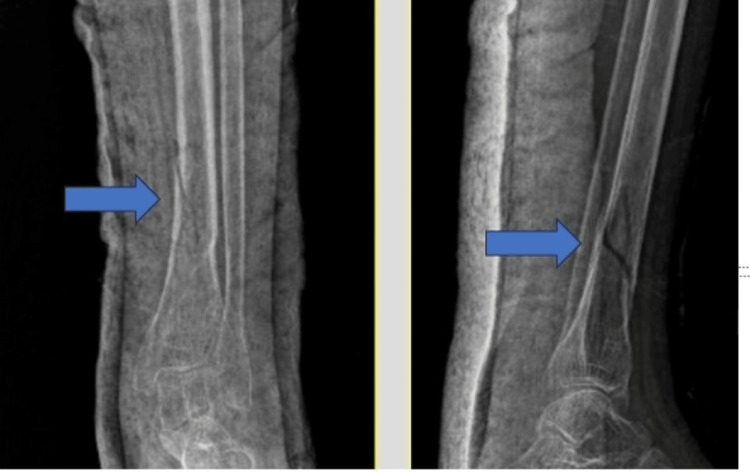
Radiograph showing extra-articular distal tibia fracture. Pointer highlighting extra-articular distal tibia fracture in anteroposterior and lateral radiographs.
